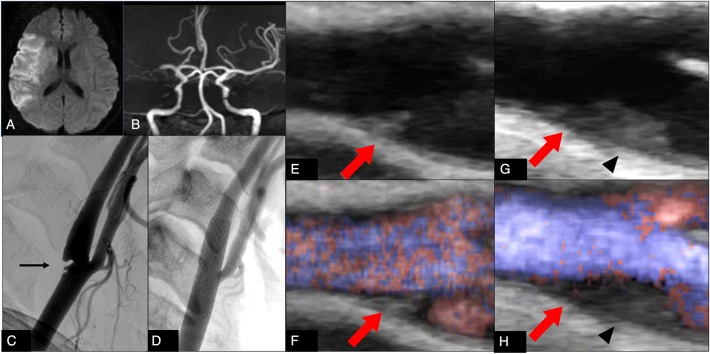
(A) Diffusion-weighted magnetic resonance imaging on admission shows a hyperintense area in the territory of the right middle cerebral artery. (B) Magnetic resonance angiography on admission shows right middle cerebral artery occlusion. (C) Digital subtraction angiography on admission shows a carotid web (arrow) at the origin of the right internal carotid artery. (D) Digital subtraction angiography after carotid artery stenting on the 32nd day shows disappearance of the web protruding into the carotid artery lumen. (E,F) Ultrasonography on the 2nd day shows a carotid web (arrow) at the origin of the right internal carotid artery (E: brightness mode, F: B-flow mode). (G,H) Ultrasonography on the 19th day shows a carotid web (arrow) and new thrombus formation on the distal portion of the carotid web (arrowhead) (G: brightness mode, H: B-flow mode).
